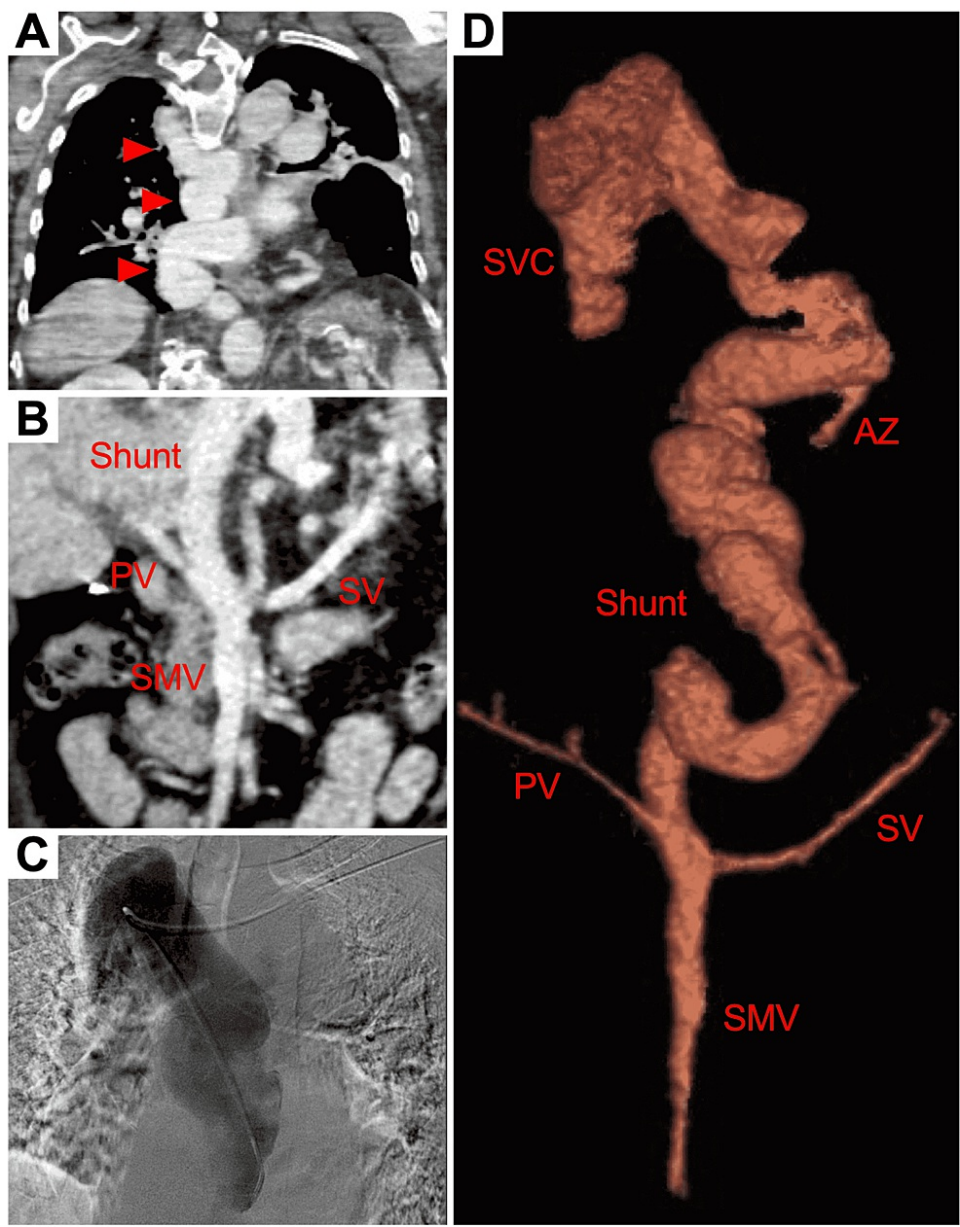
Porto-azygous shunt. A: Chest CT of the massive shunt (red arrowheads); B: CT below the diaphragm showing the superior mesenteric and splenic vein merging together to form the portal vein, which gives off the shunt; C: Angiogram of the intrathoracic shunt; D: 3D reconstruction of the shunt as it merges with the azygous vein above the diaphragm at the level of T7; SMV: Superior mesenteric vein; SV: Splenic vein; PV: Portal vein; AZ: Azygous vein; SVC: Superior vena cava.
